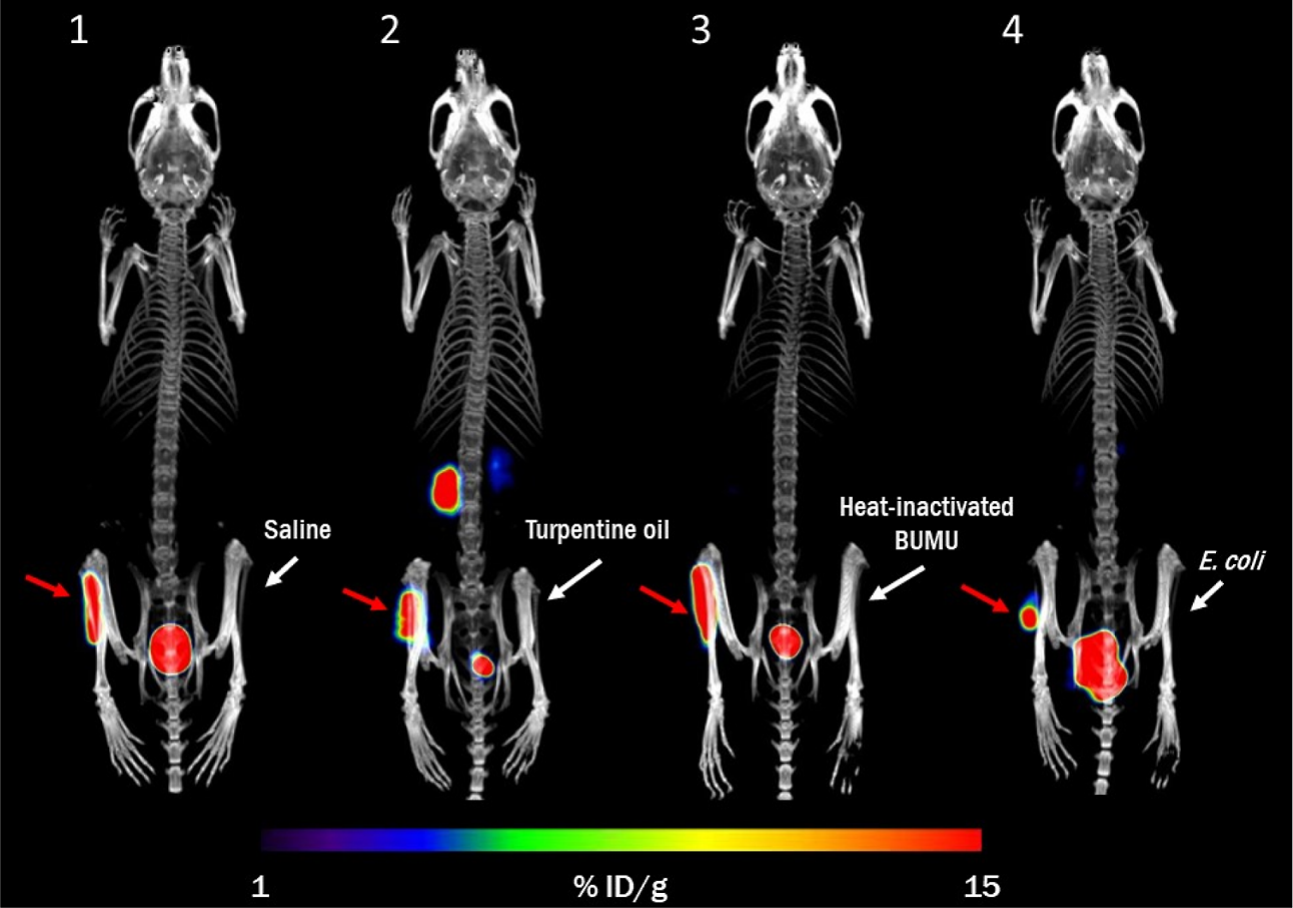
PET/CT *in vivo* imaging of [^68^Ga ]Ga-ORNB
